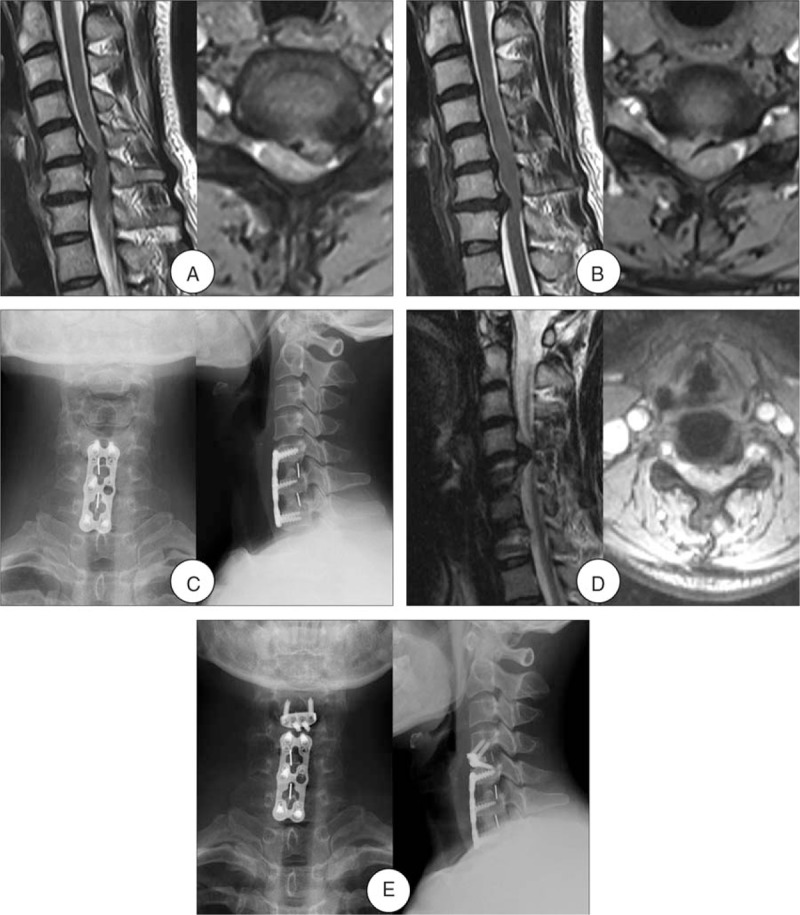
A 40-year-old woman developed one-level ASD 3 years after initial surgery. (A) and (B) Preoperative MRI of this patient shown severe compressions of the spinal cord at C5-6 and C6-7 levels. (C) Radiograph after initial surgery shown ACDF at C5-6 and C6-7. (D) MRI at 3-year follow-up shown development of ASD and spinal cord compression at C4–5. (E) Radiograph after the reoperation shown ACDF with the Zero-profile device at C4-5 and the fixed plate of the initial surgery was not removed. ACDF = anterior cervical decompression and fusion, ASD = adjacent segment disease, MRI = magnetic resonance imaging.
